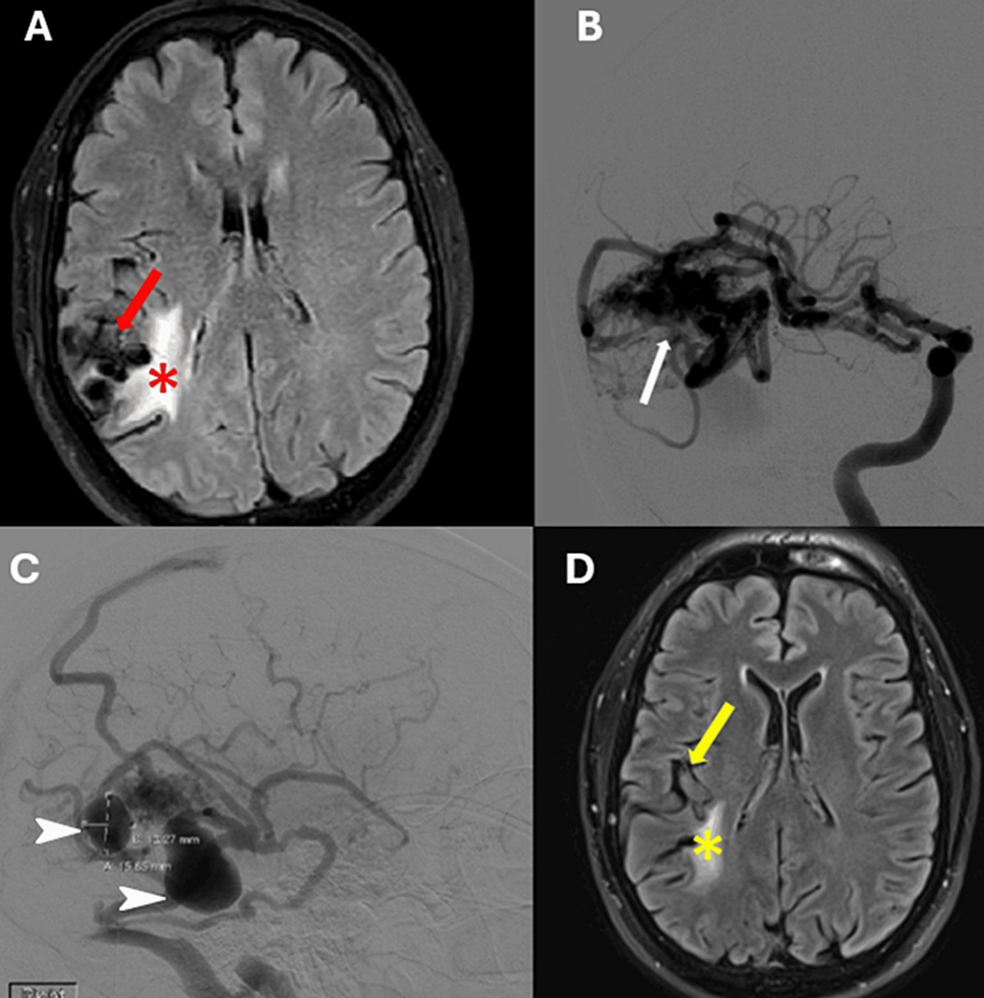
Brain MRI and Diagnostic Angiogram A: Axial FLAIR sequence of the brain showing multiple serpiginous flow-voids in the right perisylvian temporal lobe (red arrow) consistent with an arteriovenous malformation, and perilesional hyperintensity suggestive of gliosis from steal phenomena (red asterisk). B-C: DSA showing an arteriovenous malformation (B, white arrows) with main feeder from a trifurcation branch of the right MCA, and two giant varices suggestive of venous pseudoaneurysm (C, white arrowheads). D: Axial FLAIR sequence of brain MRI after one year showing right temporal lobe AVM with decreased conspicuity of parasitized vessels (yellow arrow) and decreased perilesional gliosis (yellow asterisk). FLAIR: Fluid-attenuated inversion recovery; DSA: digital subtraction angiography
